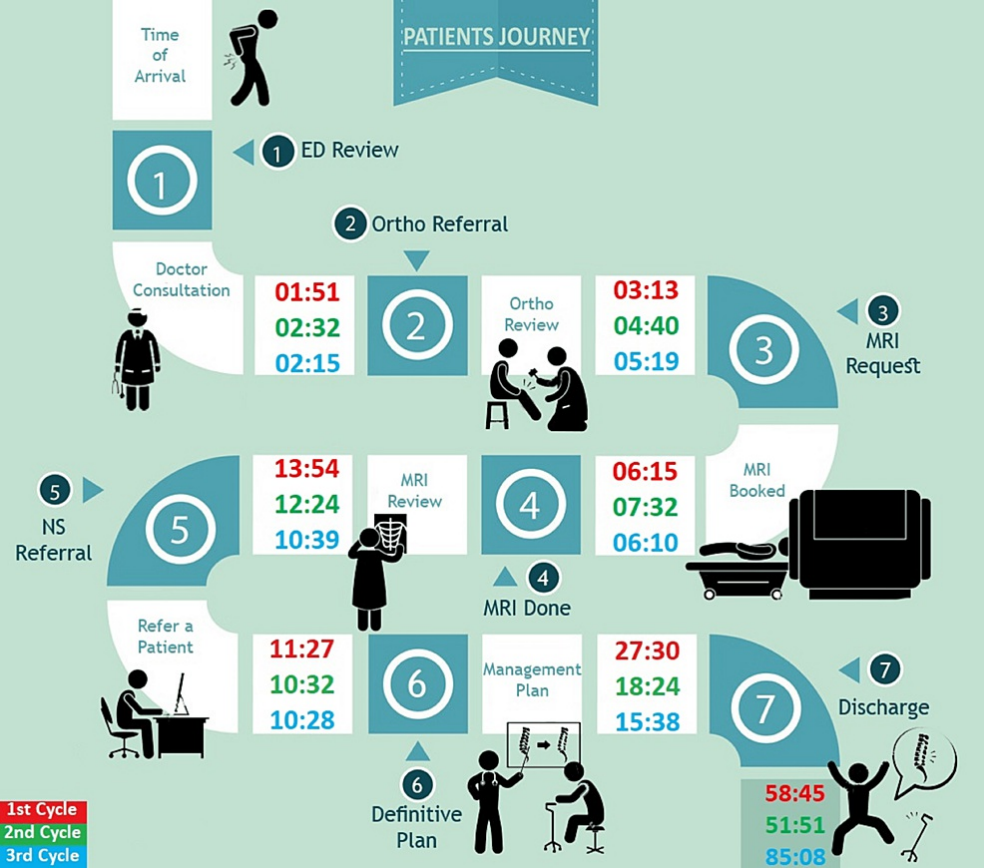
Time frame from the three audits on a patient's clinical journey through triage assessment to being discharged from the hospital with a definitive plan 1: Emergency department review; 2: Referral to the orthopedic department; 3: Request and booking of MRI; 4: Completion of MRI scan; 5: Neurosurgical referral via the electronic service; 6: Definitive plan (e.g., discharge, transfer to surgical intervention, local follow-up, conservative management); 7: Discharge from the hospital to home or another hospital in case of transfer for surgical intervention The format of the time intervals is hours:minutes Image designed by author Omer Nasim, and created by author Boulos Eskander
